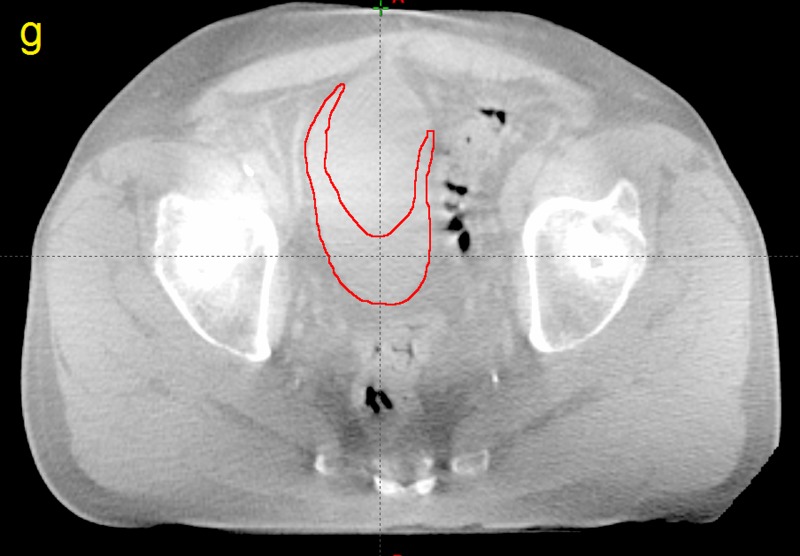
Computed tomography image taken from patient P12 g) The volume of the cone beam computed tomography bladder outside the planning target volume (red)
